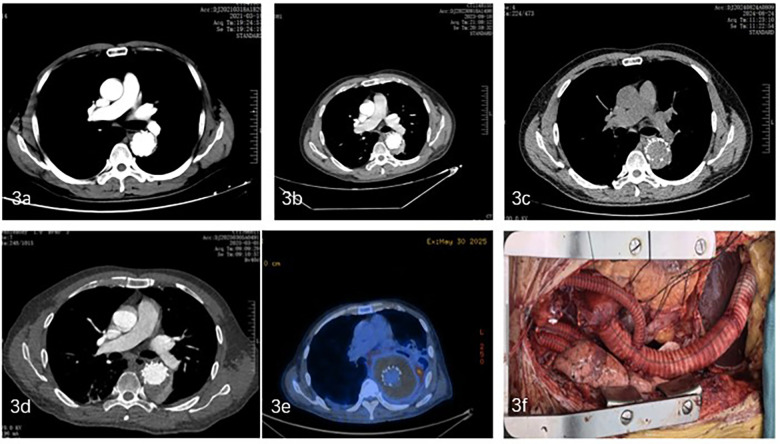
(a) three-year follow-up CTA after TEVAR. (b,c) Fluid accumulation around the stent 5–6 years after TEVAR. (d) Seven years after TEVAR, there is a significant increase in fluid accumulation around the stent graft. Seven years after TEVAR, there is a significant increase in fluid accumulation around the stent graft. (e) Seven years after TEVAR, the patient developed hemoptysis. (f) The first stage surgery involves ascending aorta-abdominal aorta artificial vascular bypass and reconstruction of the three branches of the aortic arch.
